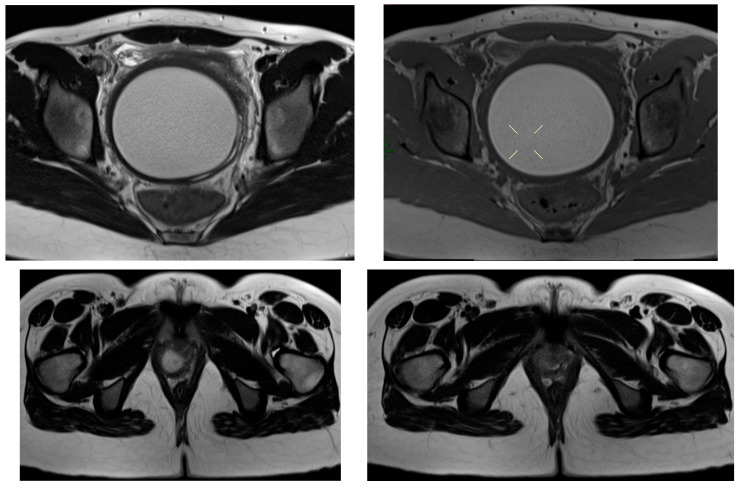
MRI pelvis in Case 3 demonstrating uterine didelphys, obstructed right hemivagina, and right renal agenesis, confirming the diagnosis of OHVIRA (Herlyn–Werner–Wunderlich) syndrome. The left kidney is solitary and normal.
